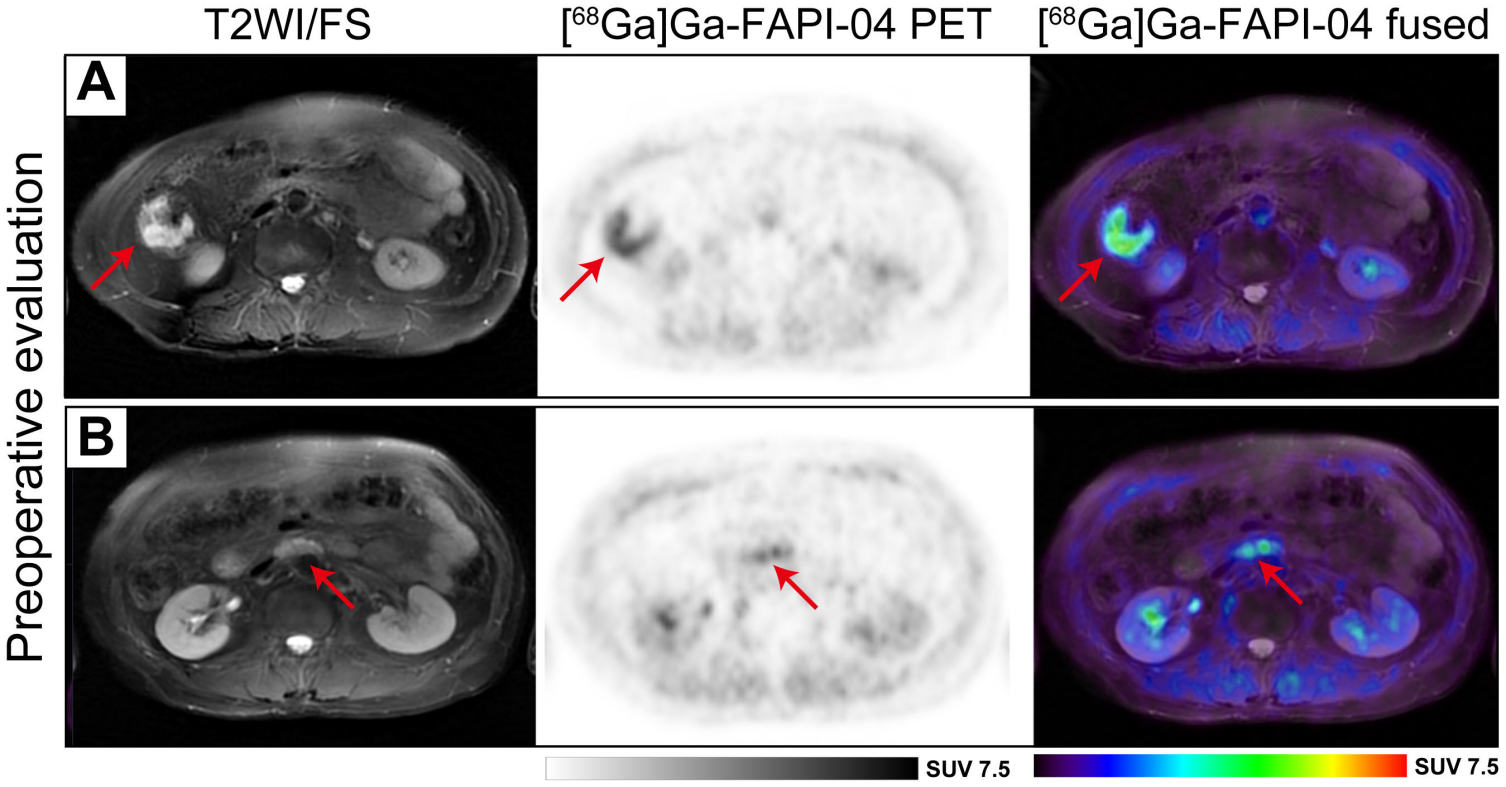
A 59-year-old male patient presented with black stool, anemia, and elevated levels of CEA and CA19-9. A colonoscopy biopsy confirmed adenocarcinoma of the ascending colon, leading to subsequent neoadjuvant immunotherapy. (A, B) [^68^Ga]Ga-FAPI-04 PET/MR revealed avid tracer accumulations (indicated by red arrows) in the ascending colon (SUVmax 4.79, SULpeak 2.66, FAPI-PTV 14.09 cm^3^, FAPI-TL 37.82) and retroperitoneal lymph node (SUVmax 4.69, SULpeak 2.49, FAPI-PTV 6.03 cm^3^, FAPI-TL 13.54). Subsequent surgical pathology revealed no residual tumor cells in these lesions.
